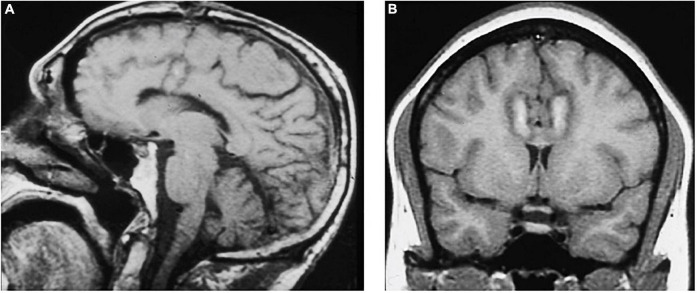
MRI-guided cingulotomy. (A) Sagittal and (B) Coronal T1-weighted MRI of acute single lesion cingulotomy.
